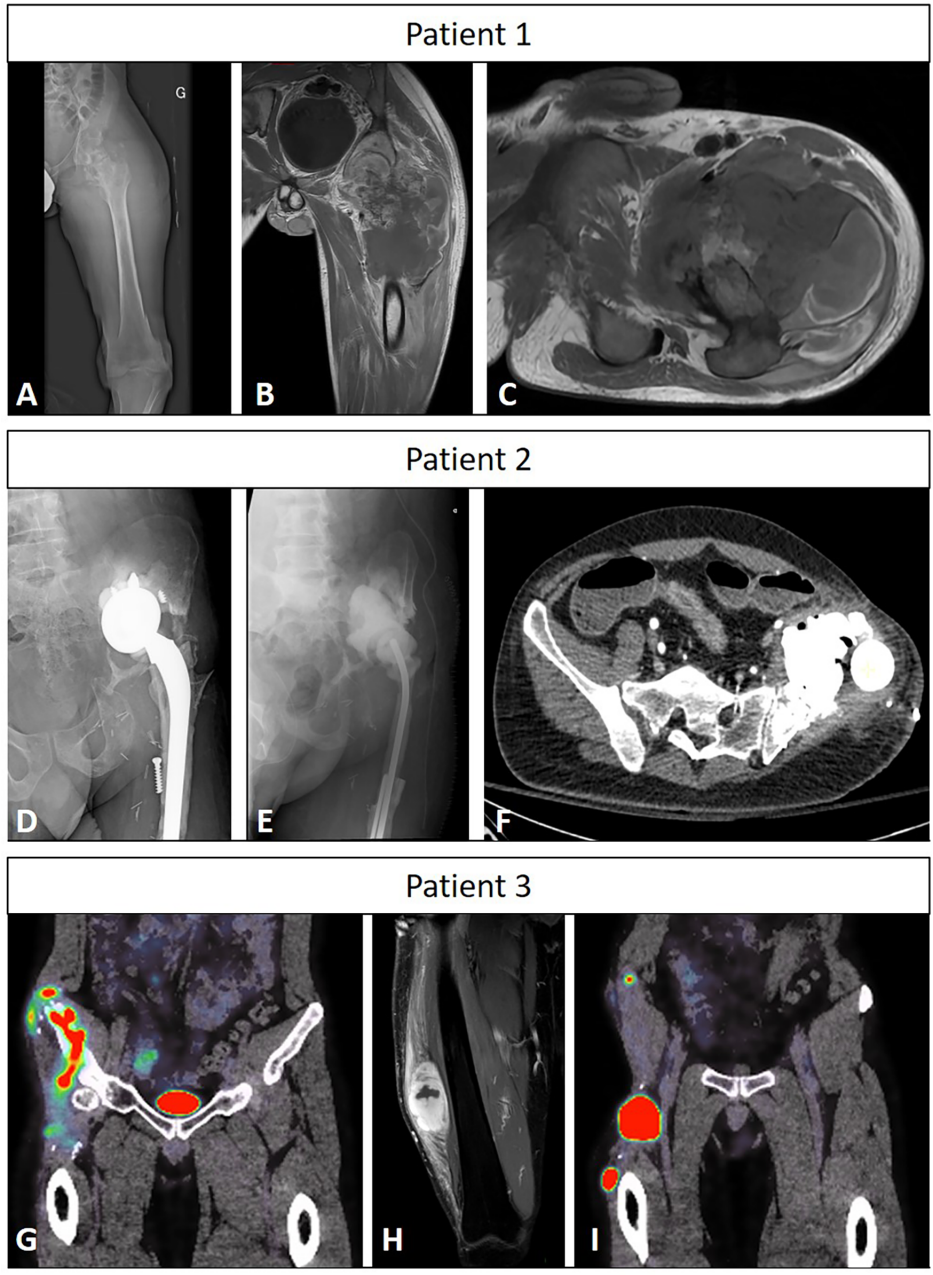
Preoperative imaging of the three patients. Patient #1: x-ray of the left hip showing a very destructive osteosarcoma (A), frontal CT-scan of the same hip showing the extension and heterogeneity of the lesion (B), and axial CT-scan around the hip of the same lesion (C) Patient #2: x-ray of the infected reconstruction of the left hip (D), x-ray of the gentamycin spacer (E), and transversal CT-scan showing the amount of cement and the spacer needed to fill the defect (F) Patient #3: Coronal CT-scan of the liposarcoma extension in the right hip and thigh (G,I) and soft-tissue magnetic resonance imaging acquisition showing another lesion on the lateral side of the thigh (H).
